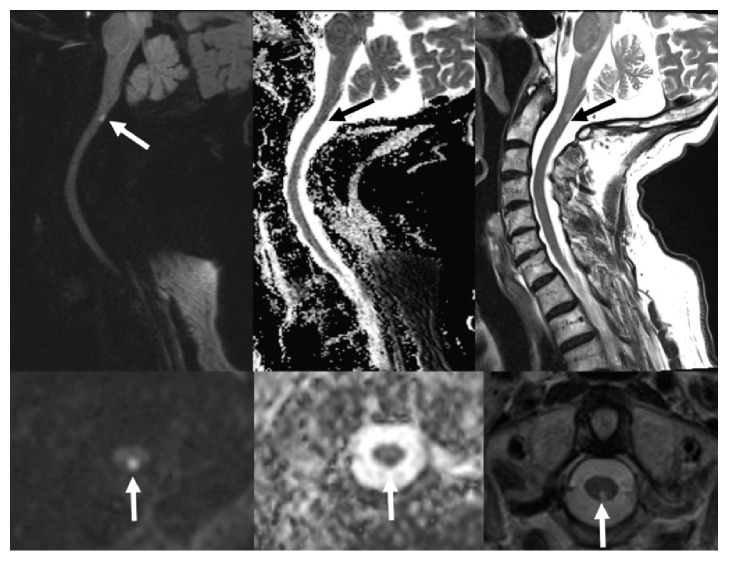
MRI Cervical spine axial and sagittal planes, (left panel) showing hyperintensity on diffusion weighted sequence, hypointensity on ADC sequence (middle panel), and hyperintensity on T2 sequence (right panel) indicating acute spinal cord infarction (arrows).
